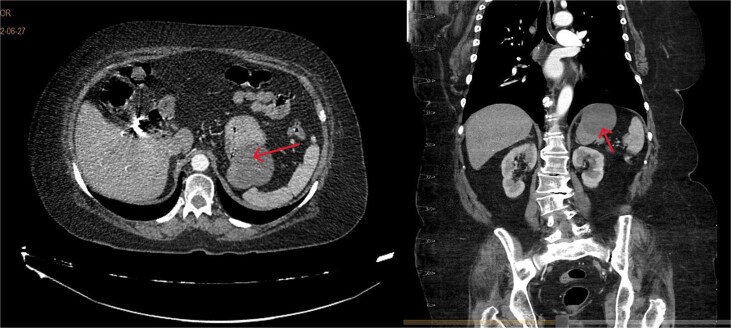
Contrast-enhanced CT, transverse, and coronal sections. Arrows: gastric duplication cyst. A slight displacement of the stomach is observed, without spleen involvement and independent from the pancreas.
